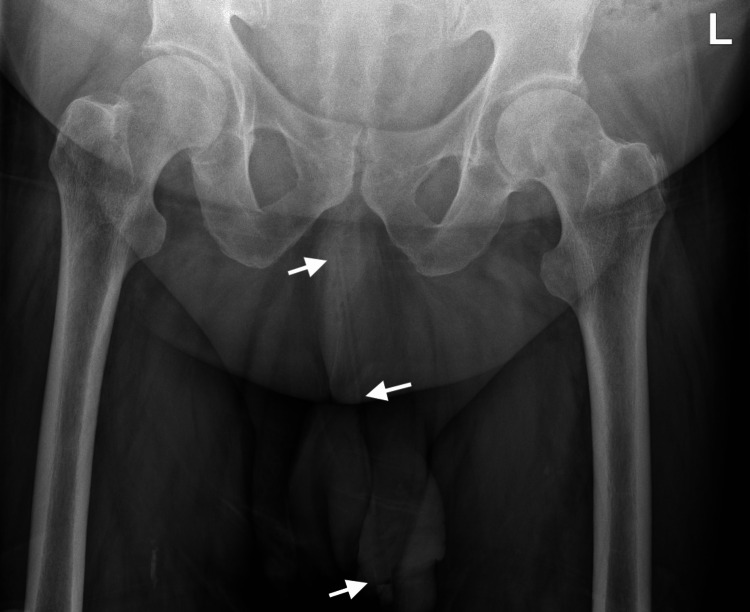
Pelvic x-ray showing a radiopaque pencil extending along the urethral tract, with the tip located proximally near the penile base
